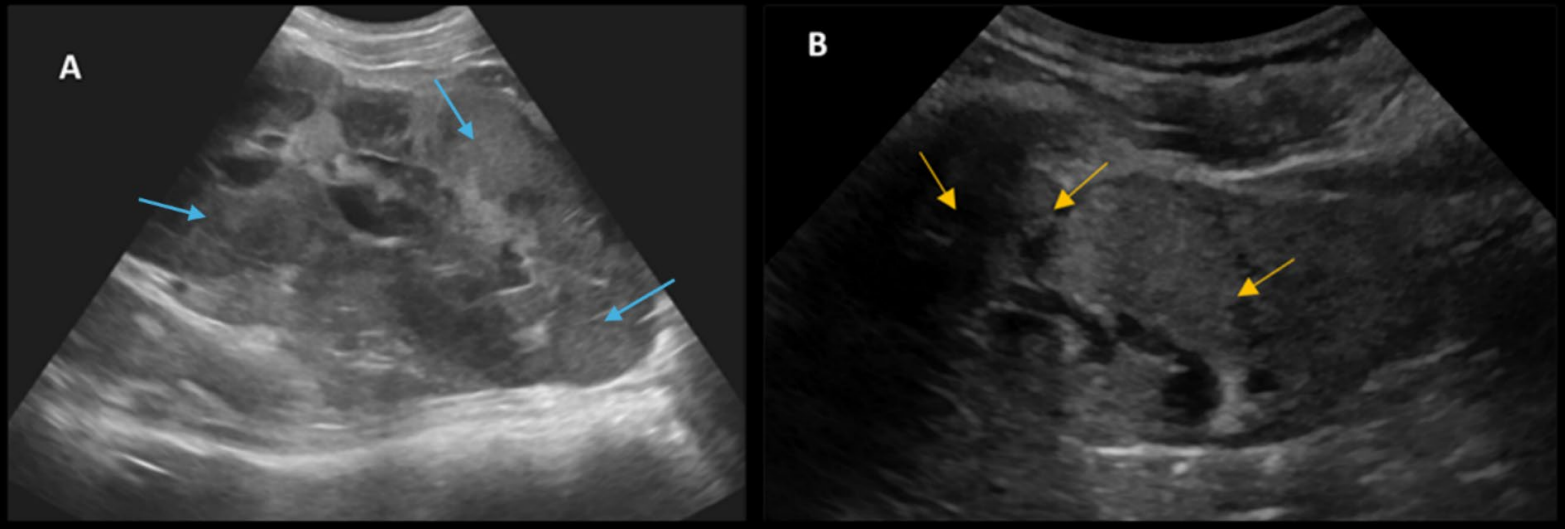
Kidney ultrasound A at diagnosis showed bilateral nephromegaly (left kidney 19 cm; right kidney 18 cm) with reduced corticomedullary differentiation and a diffuse nodular infiltrate (blue arrows) and B at 12 months after the end of therapy, with reduced corticomedullary differentiation and reduced size (left kidney 9 cm and right kidney 6.7 cm) and the presence of multiple hypoechogenic scar tracts (yellow arrows)
